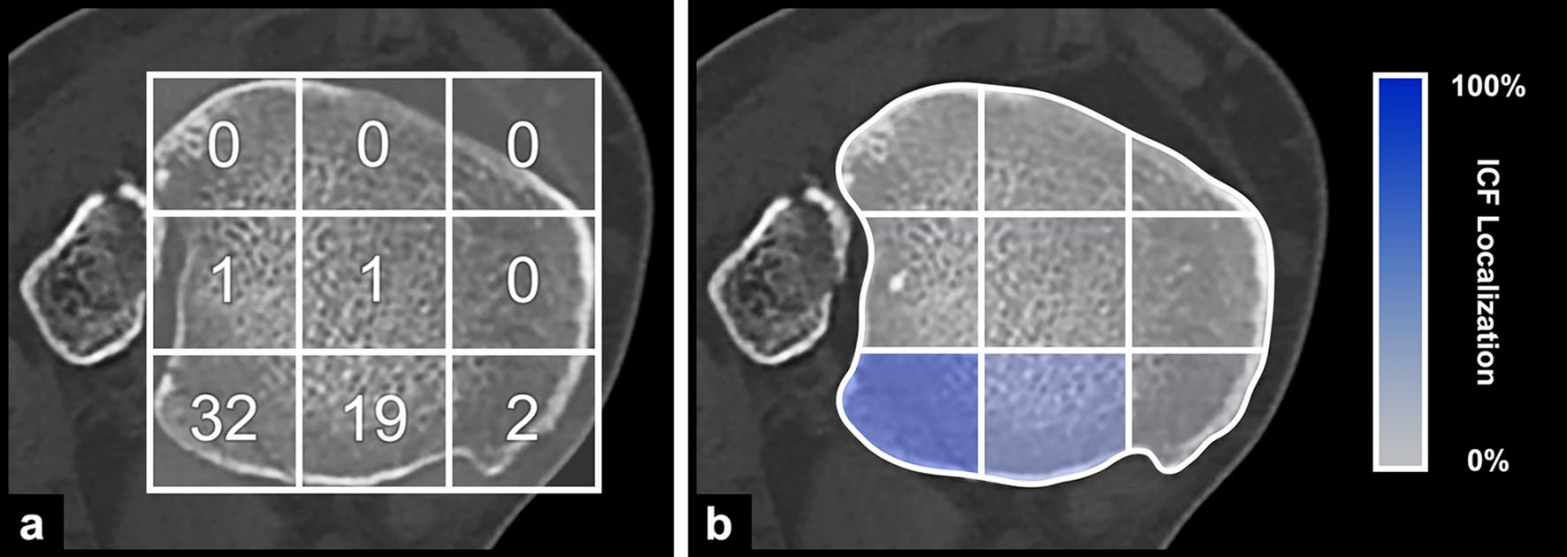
Location of the intercalary fragments within the nine regions of the CT scan axial plane in a absolute numbers b percentages
